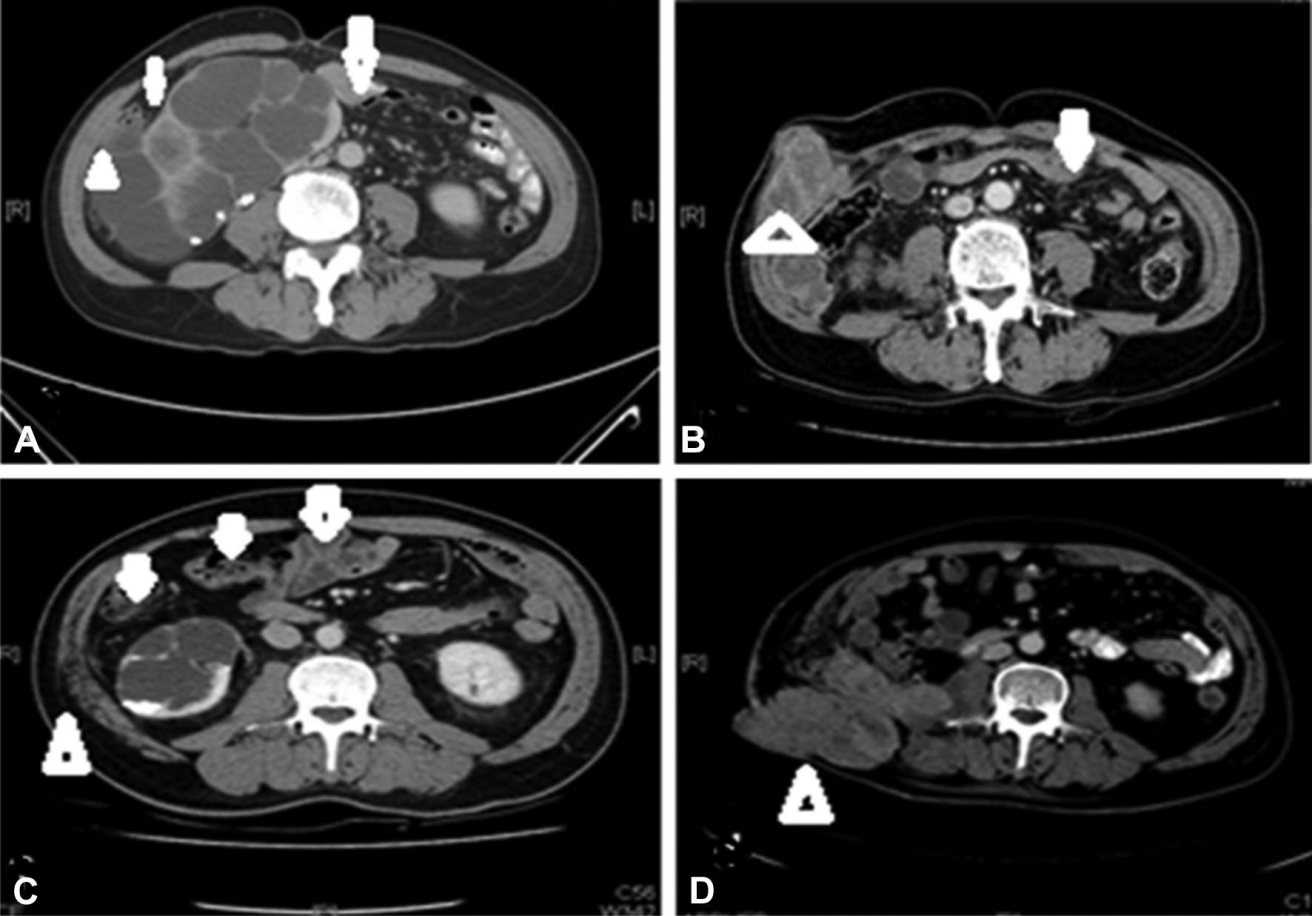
CT scans showing peritoneal thickening of renal pelvis carcinoma. Axial views of Case 1 (a, b) and Case 2 (c, d) before nephroureterectomy, all showing peritoneal thickening with lateral abdominal wall invasion. b Axial view showing tumor progression with an obvious right lateral abdominal wall mass following surgery and chemotherapy. Arrows indicate a thickened or dirty peritoneum. Arrowheads indicate abdominal wall invasion lesions
